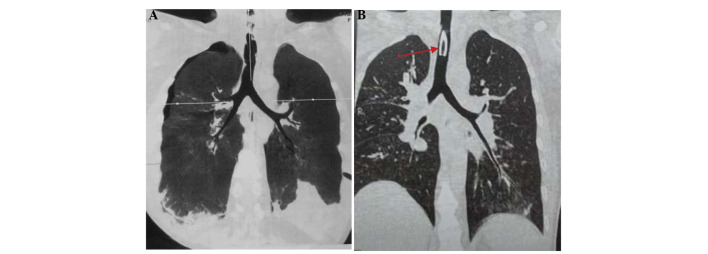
(A) Tracheal 3D CT scan images of the patient on day 13 after treatment showing airway mucosal damage and pneumothorax. (B) Tracheal 3D CT scan images of the patient on day 33 after treatment. Red arrows indicate metal trachea cannula. 3D CT, three-dimensional computed tomography.
